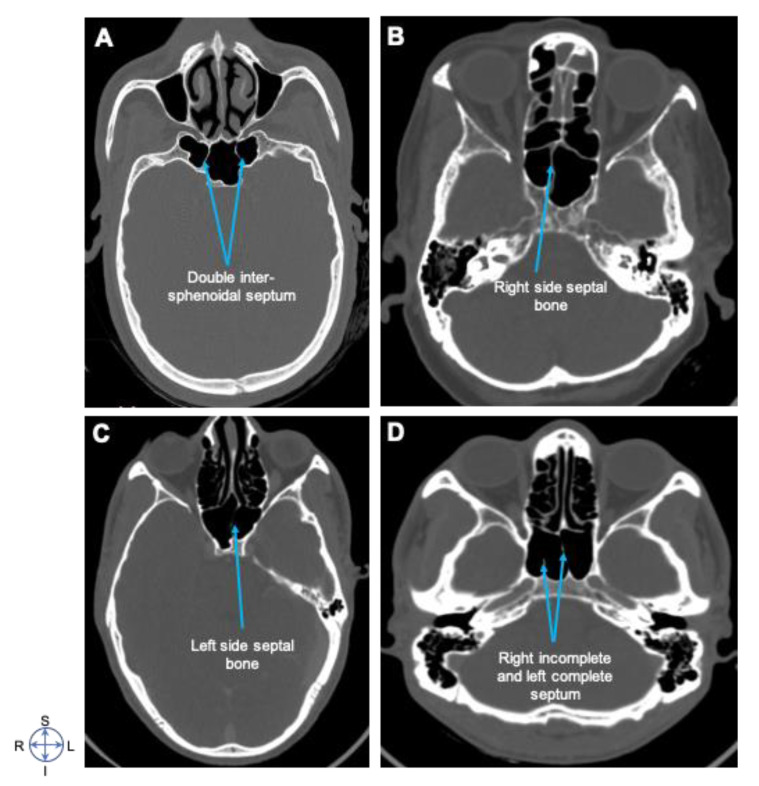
Septation of the sphenoid sinus. Axial CT images showing (A) Double inter-sphenoidal septum, (B) Right side septal bone, (C) Left side septal bone, and (D) Right incomplete and left complete septum. Note that almost 90% of the patient population who participated in this study showed single septation, whereas 1% without septation. Image orientation: S, superior; I, inferior; R, right side; L, left side.
